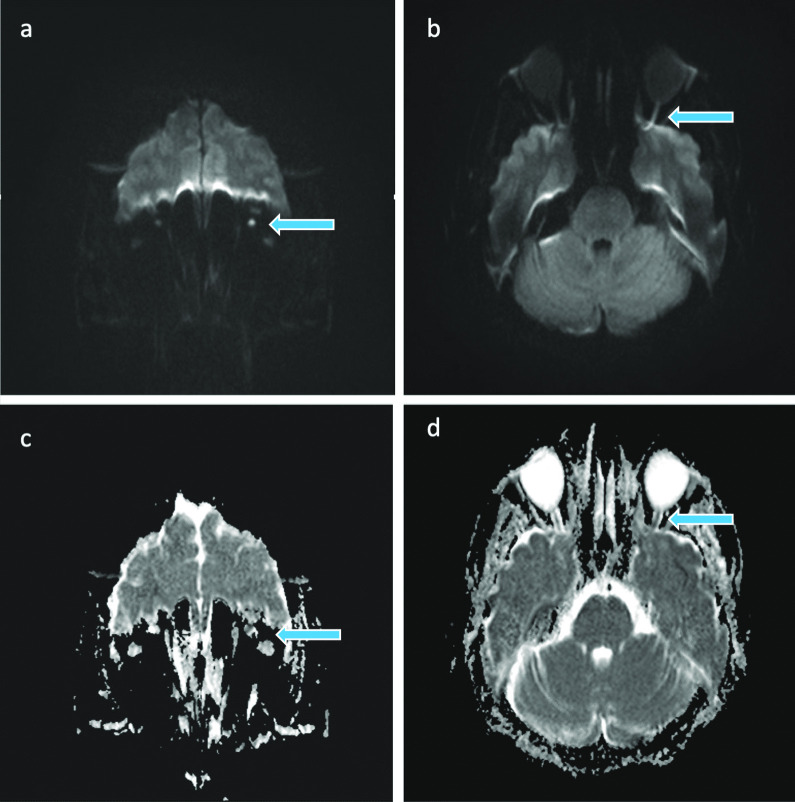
Initial MRI of the brain. Coronal (a) and axial (b) diffusion weighted imaging (DWI) b = 1000 images and corresponding apparent diffusion coefficient (ADC) maps (c, d) depicting high b = 1000 DWI signal and low ADC in the left optic nerve of our patient at presentation
